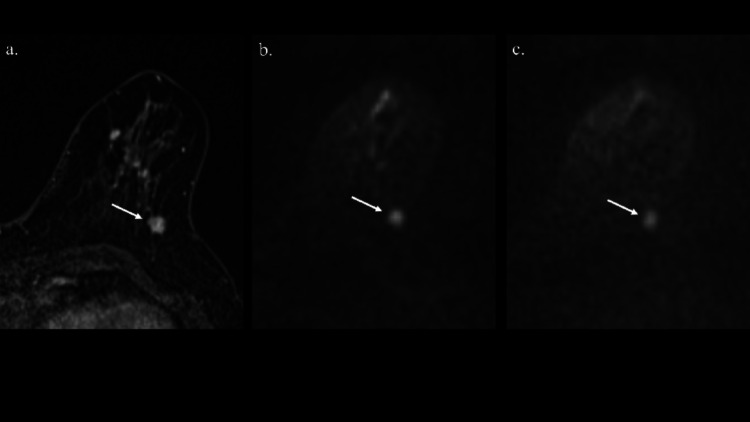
A 70-year-old woman underwent preoperative MRI for invasive ductal carcinoma in the right breast. On early-phase DCE-MRI, an 8.1-mm MRI-detected mass lesion was observed in the left breast (contralateral to the known breast cancer) (a, arrow) and assessed as BI-RADS category 4. On both DWI (b, DWI_800_ and c, DWI_1500_), the lesion was clearly visualized with high contrast against the fibroglandular tissue (b, c, arrow). Both readers assigned a DWI visibility score of 4 on both DWI. On MRI-targeted ultrasonography, the lesion was depicted as a 6.6-mm mass and was diagnosed as invasive ductal carcinoma by ultrasound-guided core needle biopsy (DWI true-positive)
